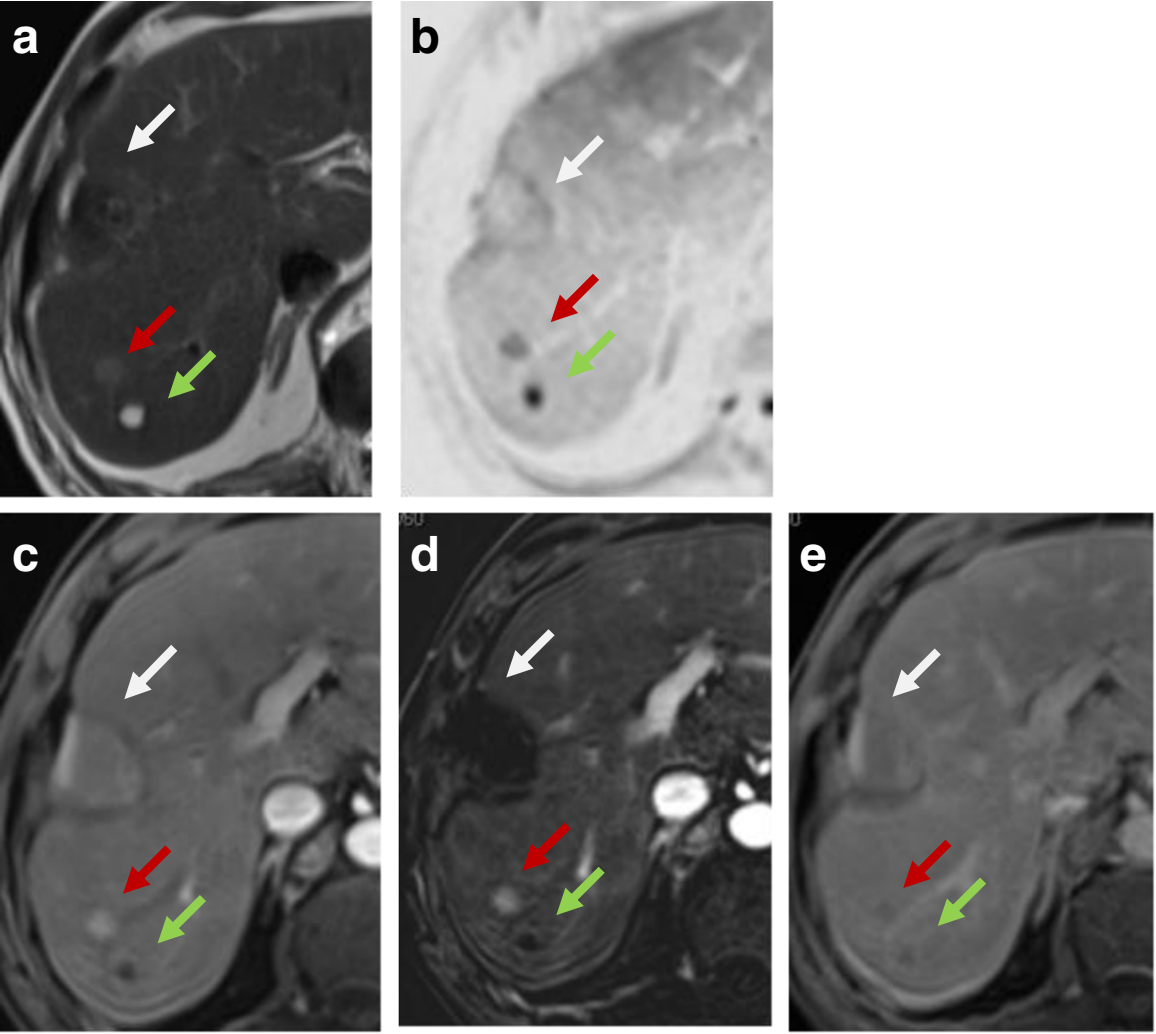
Hepatic focal lesion after RFA. a T2-WI shows a well-defined treated subcapsular focal lesion (white arrow). Two other smaller lesions are seen posteriorly; one is of an intermediate signal (red arrow) and the other is of a high signal (green arrow). b Inverse of DWI shows no restriction of the treated lesion (white arrow), yet shows restriction of the other two lesions. ADC (not shown) shows T2 shine through of the posterior lesion (green arrow) with true restriction of the anterior lesion (red arrow). c Enhanced T1-WI in the arterial phase shows a high signal of the treated lesion that can be misinterpreted as an arterial hyperenhancing focal lesion. Note the enhancement of the anterior lesion and non-enhancement of the posterior small lesions. d Subtracted image shows no appreciable enhancement of the treated lesion and posterior lesion, yet shows definite enhancement of the red-arrowed lesion. e Delayed T1-WI shows washout of the red-arrowed lesion. Final diagnosis is LR-TR nonviable, new small HCC, and small hepatic cyst
